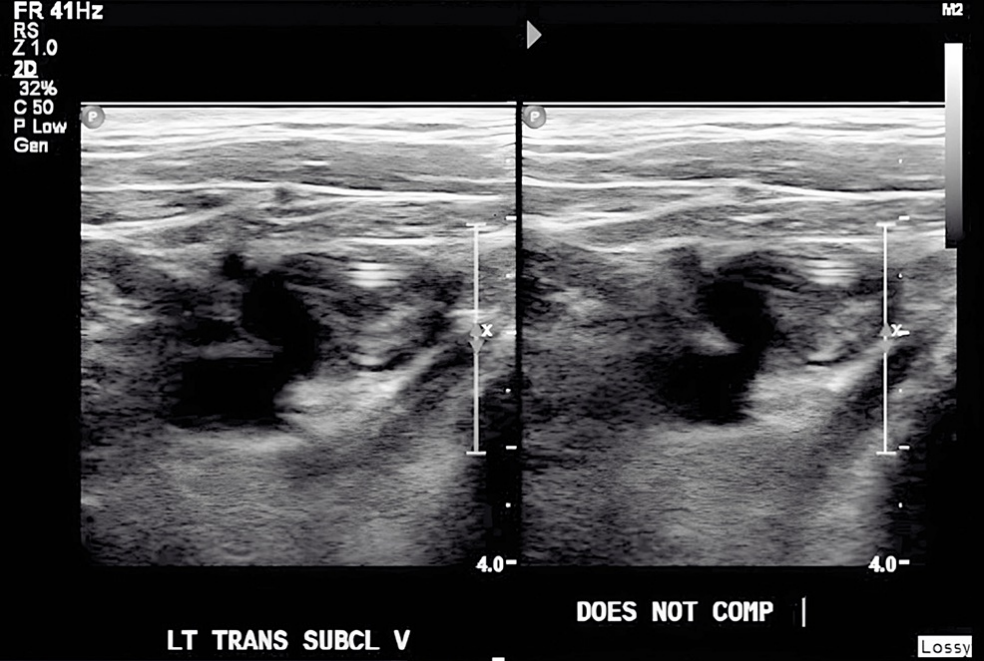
Venous Doppler showing thrombosis in the left subclavian vein
